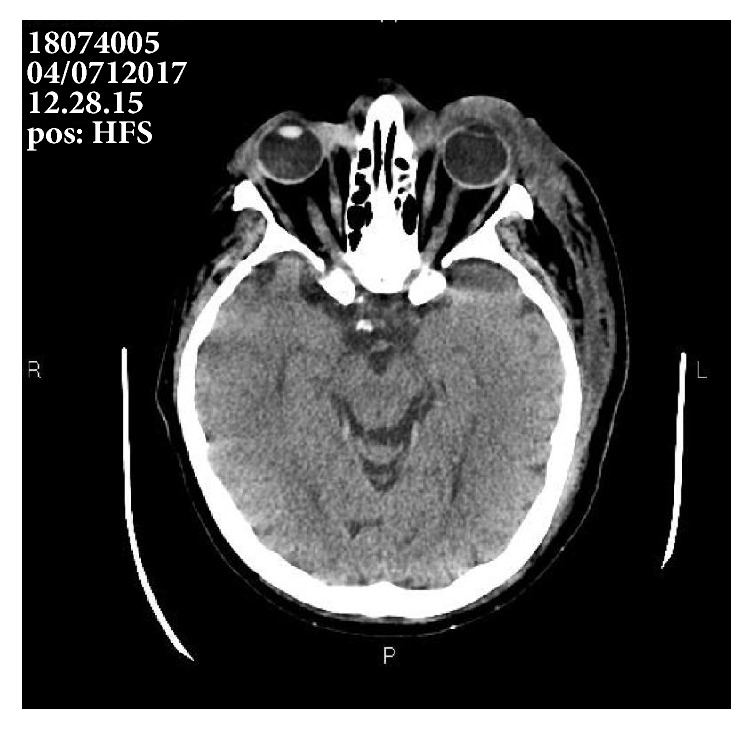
Computed tomography showing soft tissue swelling.
